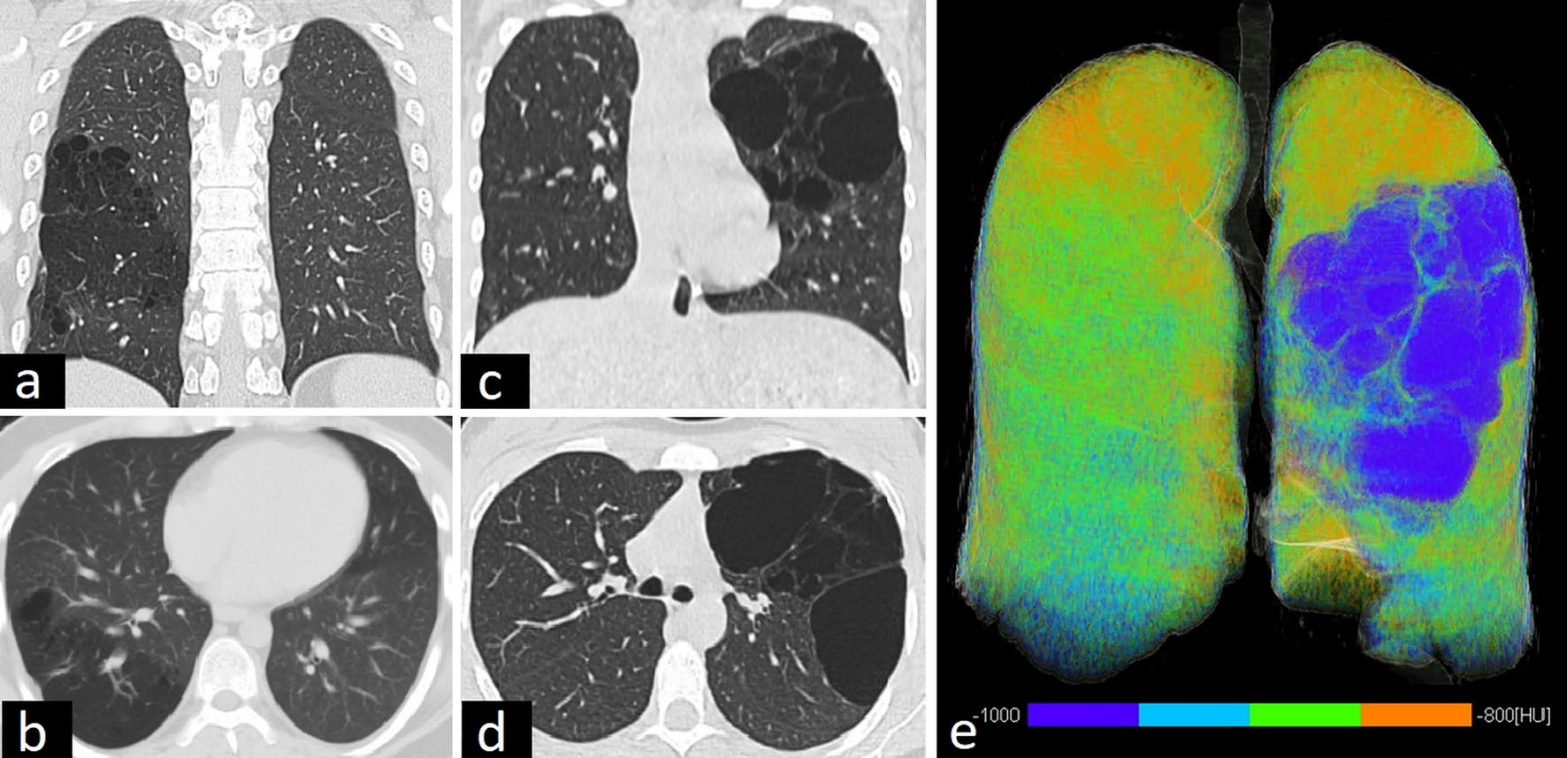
CT findings of congenital pulmonary airway malformation (CPAM) in two adult patients. Cysts < 2 cm in type 2 CPAM (a, b) and > 2 cm in type 1 CPAM (c, d) are seen on coronal and axial CT images. Quantitative 3D reconstruction image (e) depicts hyperinflation and lower lung density in the patient with type CPAM 1 (blue area)
